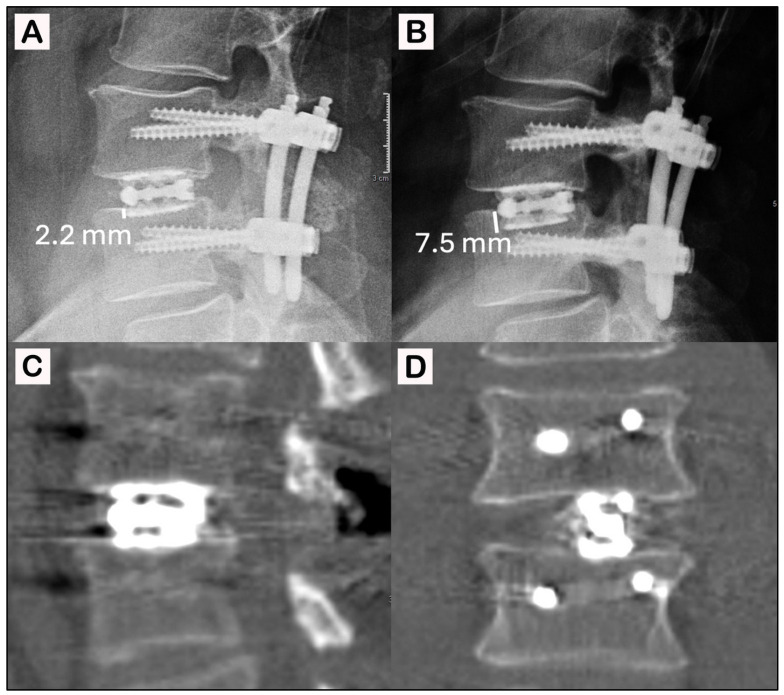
Progressive Interbody Cage Subsidence Over 1 Year; Early Assessment Limited by Intraoperative CT Streak Artifact.* (A) Immediate postoperative lateral radiograph demonstrates mild subsidence of the interbody spacer. (B) Lateral radiograph at approximately 12 months shows marked progression with greater endplate indentation and loss of disc height. (C) Sagittal and (D) coronal reformats of the intraoperative CT obtained after instrumentation; streak artifact from the hardware obscures the endplate–implant interfaces, making early subsidence difficult to appreciate on intraoperative imaging. Representative patient: 67-year-old male, BMI 30.52 kg/m^2^, operated level L3–4. *Abbreviations:* BMI = body mass index; CT = computed tomography; L = lumbar.
